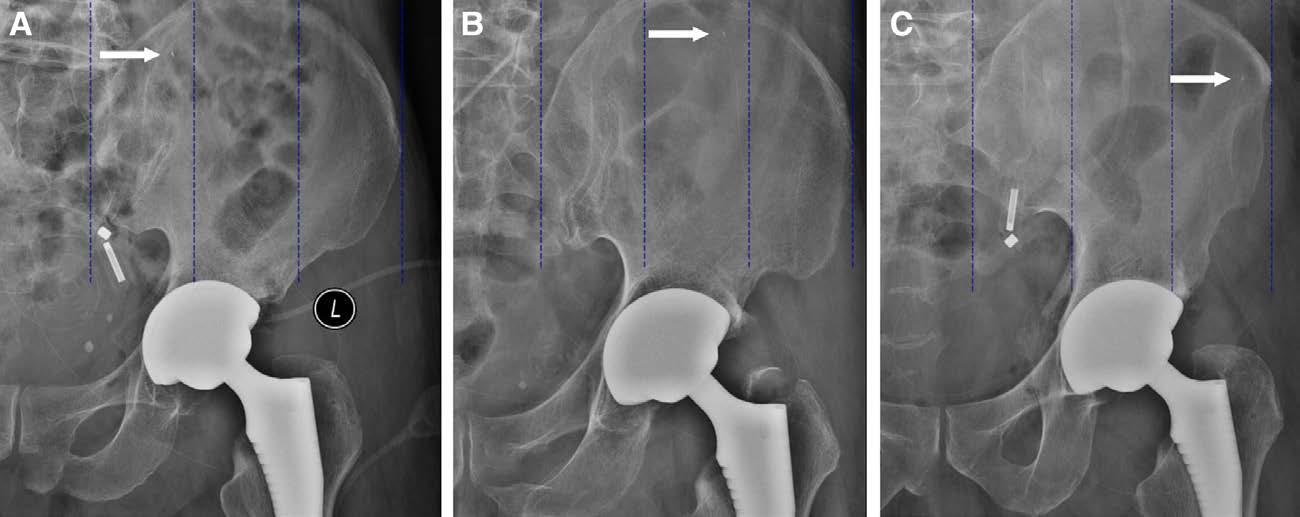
Postoperative X-ray confirmation of catheter placement. Postoperative X-ray images showing the catheter placement relative to the preoperative position. The black arrow indicates the tip of the catheter, confirming that there was no displacement within the fascia iliaca compartment. This verification ensured consistent analgesic delivery throughout the postoperative period.
